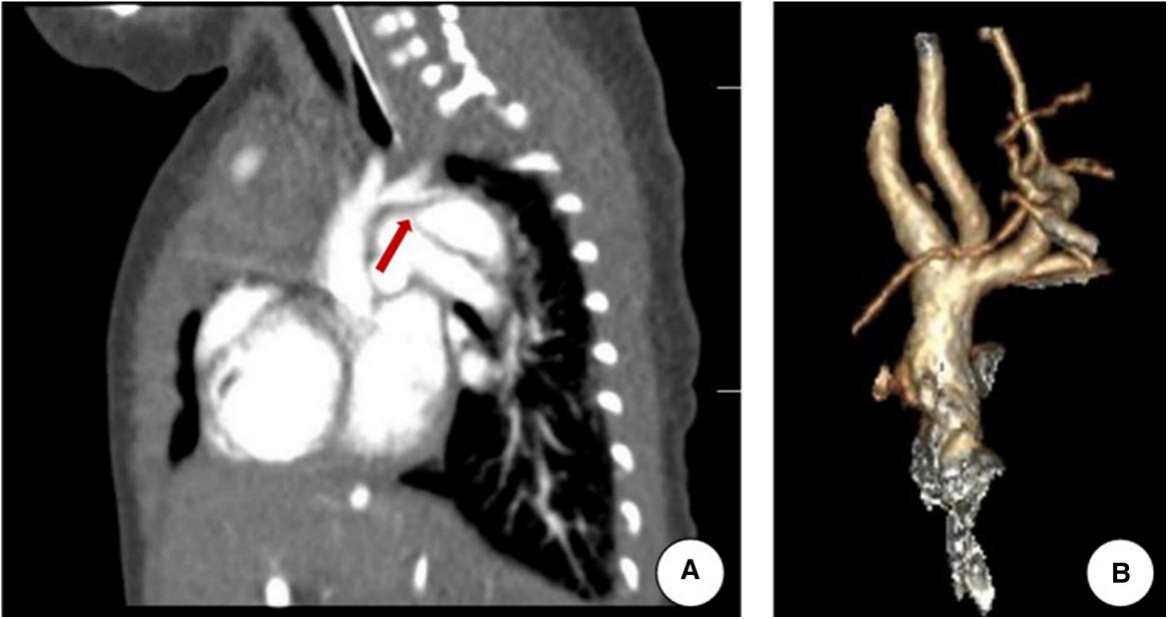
Sagittal and 3D reconstruction images of the computed tomography angiography. (*A*) Sagittal image. (*B*) 3D reconstruction; red arrow indicates arch hypoplasia.
